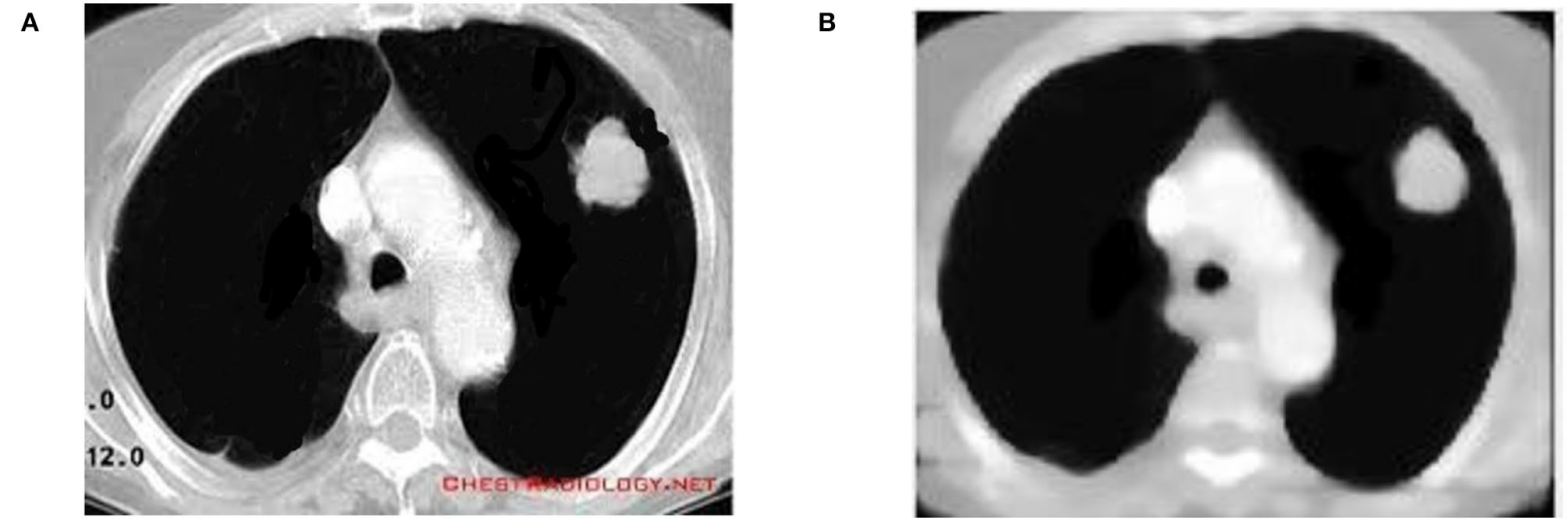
(A) Input CT image. (B) Filtered output.
